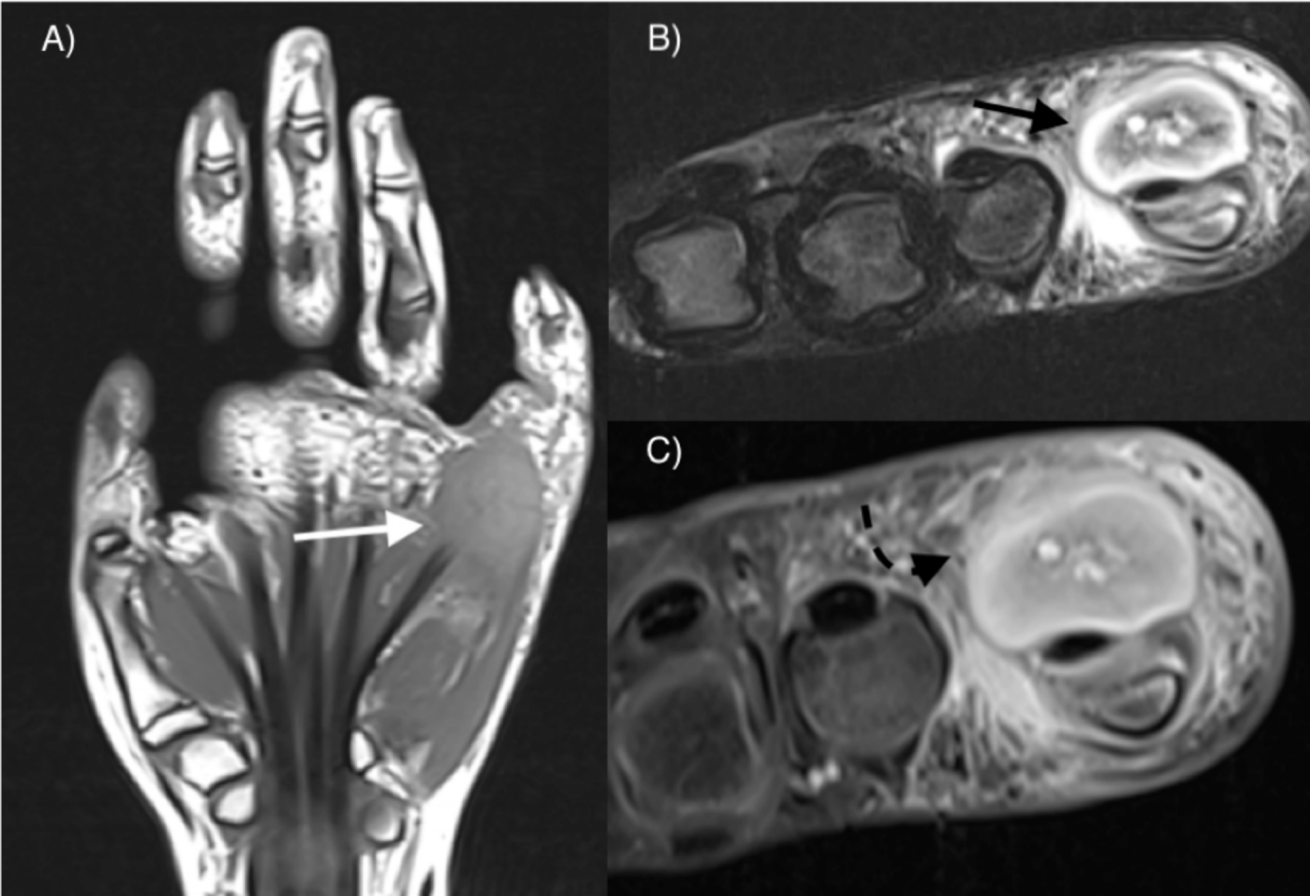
MRI findings A) coronal T1-weighted demonstrates a mass which is homogeneously isointense to muscle (white arrow); B) axial T2-weighted FS demonstrates heterogeneous signal, hyper-intense in the periphery (black arrow); C) contrast-enhanced axial T1-weighted FS image demonstrates rim-like enhancement (curved discontinuous arrow), as well as enhancement of some central foci MRI: magnetic resonance imaging; FS: fat suppression
